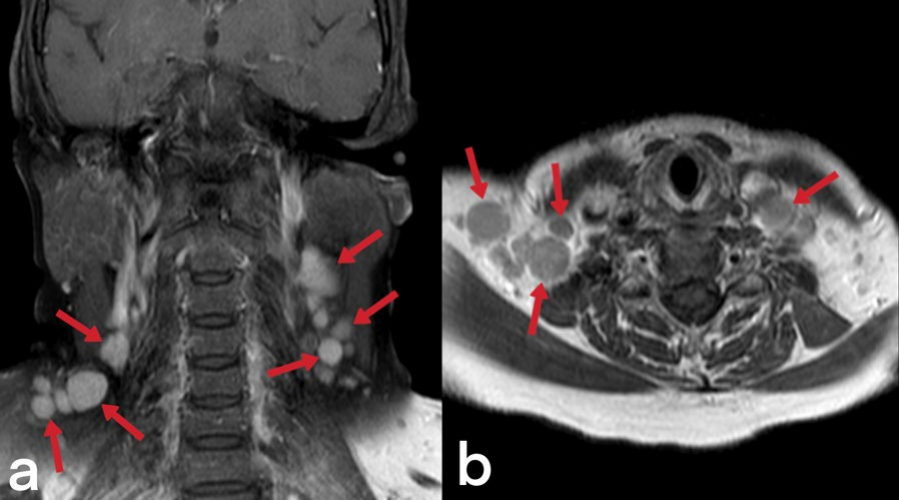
Contrast-enhanced T1 weighted coronal (a) and axial T1 weighted (b) MR images of a 44-year-old female demonstrate multiple lymphadenopathies in the bilateral cervical chains and right supraclavicular region. Multiple intraabdominal lymphadenopathies are also noted (not shown)
